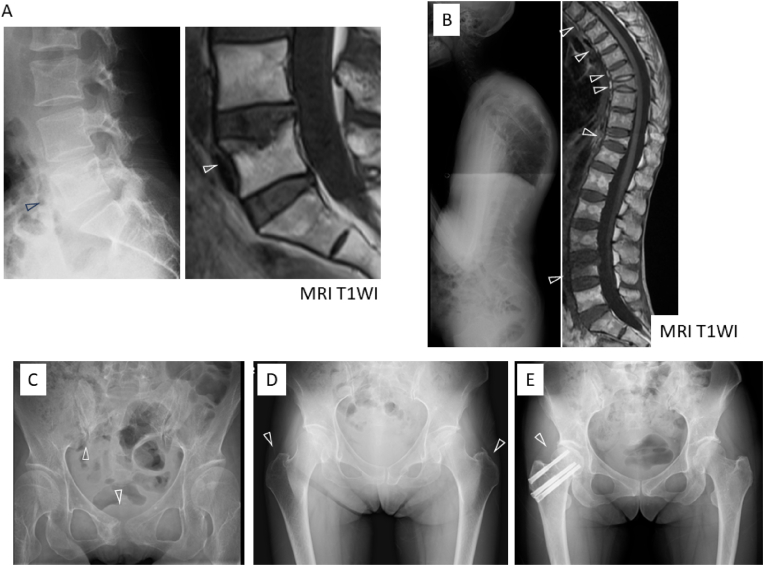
The images of past fractures. X-rays and T1 weighted images (T1WI) of magnetic resonance imaging (MRI) A: case 1, An L5 compression fracture. B: case 2, Multiple thoracolumbar compression fractures (T3, 5, 7, 8, 11, L4). C: case 3, Right pubic and sacral fractures. D: case 4, Bilateral femoral greater trochanteric fractures. E: case 5, A right femoral neck fracture.
